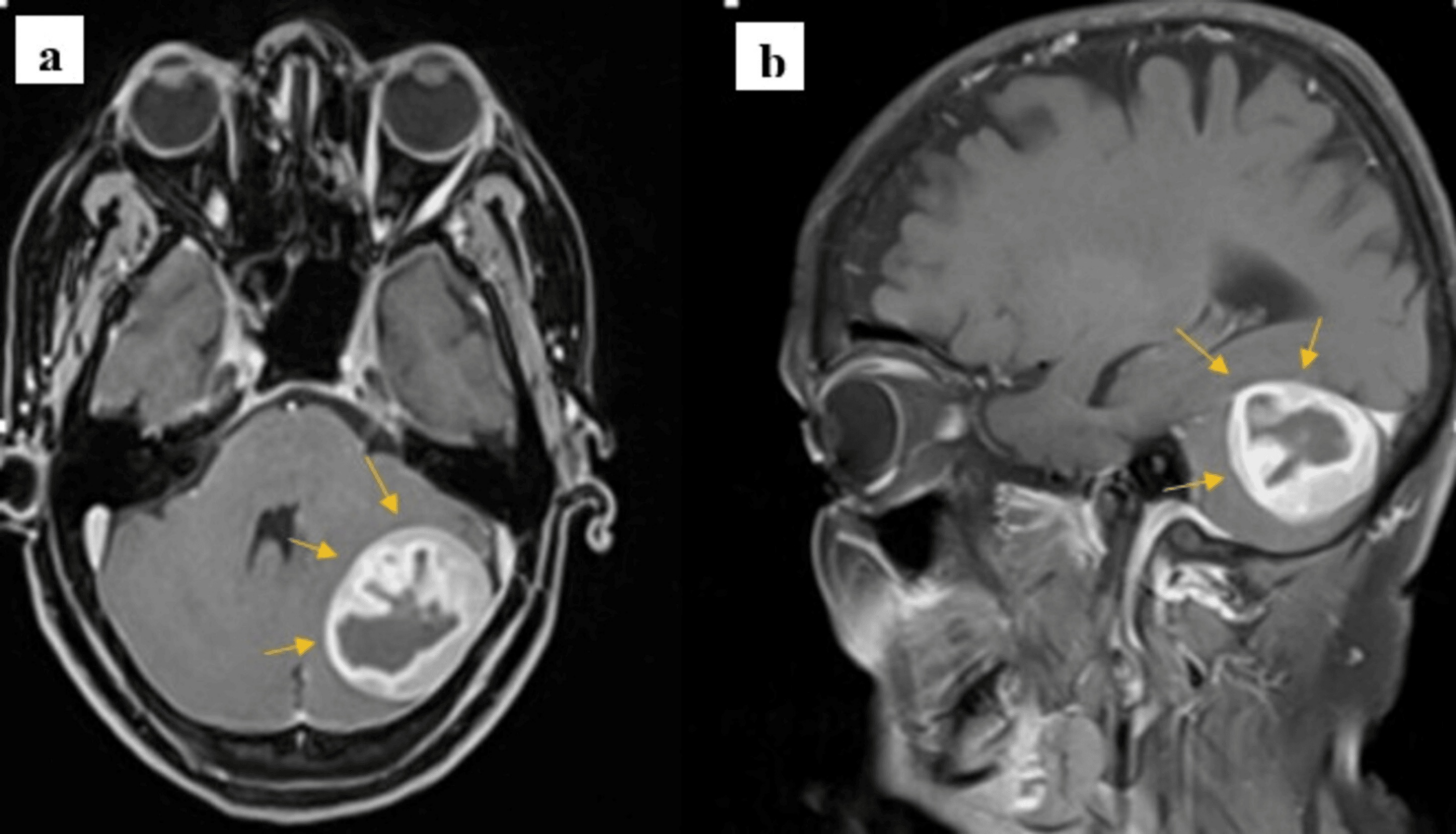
(a,b) Axial and sagittal post-contrast MRI demonstrates avid heterogeneous peripheral enhancement (yellow arrow) with central non-enhancing areas, likely necrotic. Adjacent dural enhancement (dural tail) is noted. Mild adjacent peripheral edema is also present.
